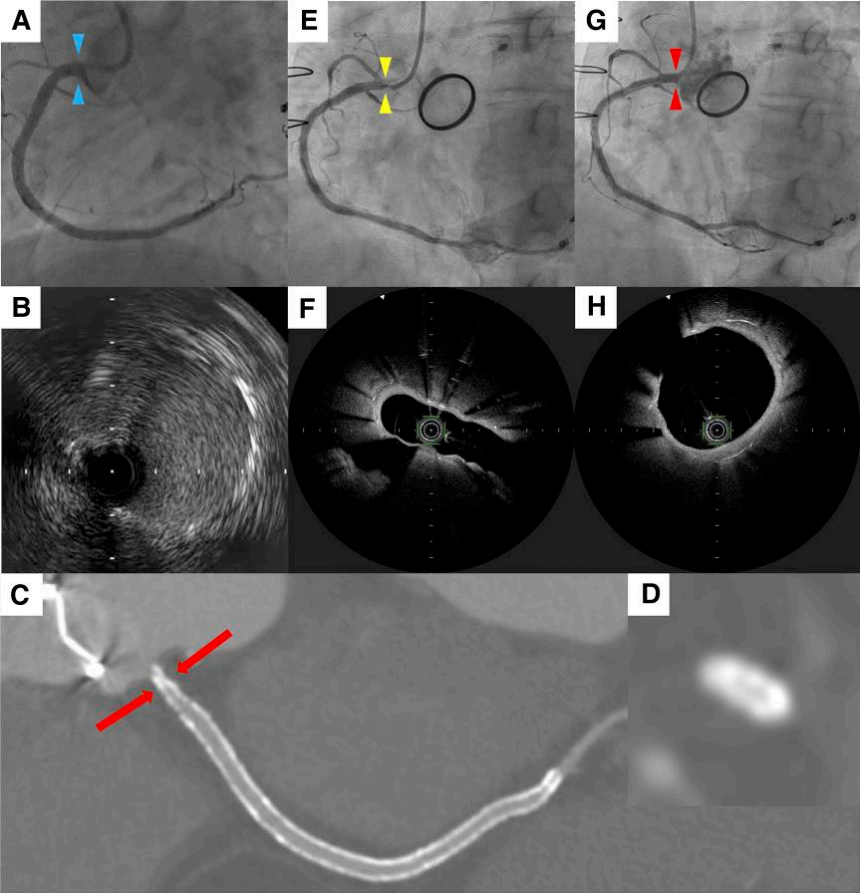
A 71-year-old woman with effort angina was admitted to our institution. She was previously diagnosed with hypertension and hyperlipidaemia. Eight years prior to admission, she underwent percutaneous coronary intervention (PCI) of the right coronary artery (RCA) ostium with a drug-eluting stent (Promus Premier 3.5/38 mm, Boston Scientific, Natick, MA, USA) (*Panels A* and *B*). Three years ago, she developed heart failure due to severe aortic regurgitation and underwent surgical aortic valve replacement (SAVR; 18 mm ATS AP valve, ATS Medical Inc., Minneapolis, MN, USA) under cardiopulmonary bypass. Due to frequent effort angina, we performed the coronary computed tomography angiography, revealing luminal narrowing due to severe stent deformation at the proximal RCA (*Panels C* and *D*). Invasive coronary angiography showed angiographically equivocal stenosis of the RCA ostium (*Panel E* and Supplementary data online, *Video S1*). Optical frequency domain imaging (OFDI) (FastView, Terumo, Tokyo, Japan) verified stent deformation at the RCA ostium without intimal proliferation (*Panel F* and Supplementary data online, *Video S2*). The patient underwent PCI of the RCA ostium using paclitaxel-coated balloon (SeQuent Please, 4.0/20 mm, B. Braun Melsungen, Germany) (*Panel G*). The OFDI confirmed re-expansion of the deformed stent (*Panel H*).
